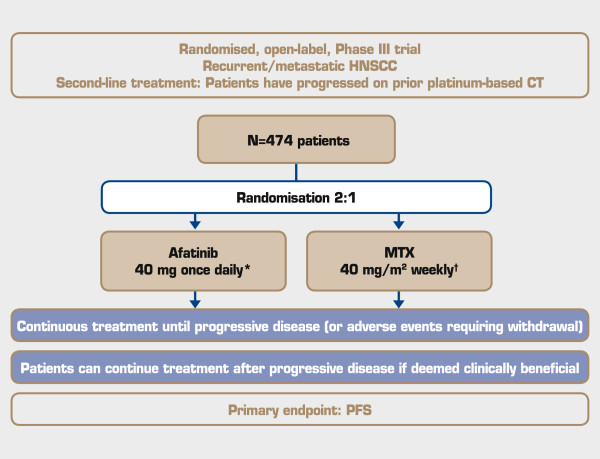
Trial design. *Dose escalation to 50 mg once daily and/or reduction to 40, 30, then 20 mg once daily. ^†^Dose can be escalated to 50 mg/m^2^ weekly and/or reduction to 40, 30, then 20 mg/m^2^ weekly. HNSCC = head and neck squamous cell carcinoma; CT = chemotherapy; MTX = methotrexate; PFS = progression-free survival.
